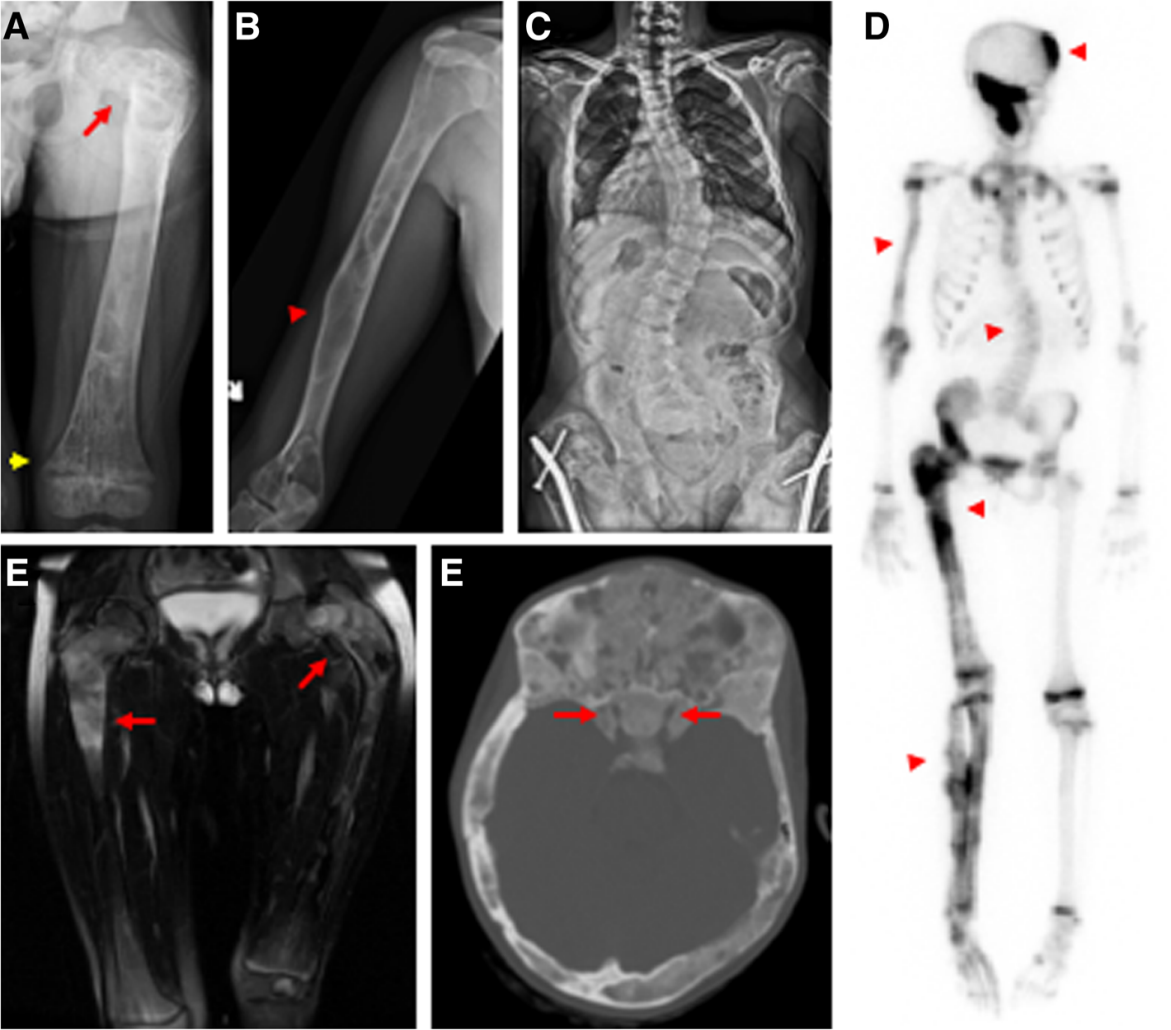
Representative radiographic features of fibrous dysplasia. a Femoral X-ray demonstrating diffuse involvement with fibrous dysplasia and a coxa vara (“shepherd’s crook”) deformity (red arrow). Note the irregular appearance of the distal femoral metaphyses (yellow arrowhead) resulting from FGF-23-mediated rickets. b Humeral X-ray demonstrating characteristic features of fibrous dysplasia, including homogenous “ground glass” appearance and cortical thinning. Bowing has occurred at a previously fractured site in the midshaft (red arrowhead). c X-ray from a patient with diffuse spinal FD and resulting thoraco-lumbar scoliosis. Note the presence of bilateral intramedullary femoral rods. d Technetium-99 scintigraphy scan showing increased tracer uptake in areas of fibrous dysplasia, including the skull, spine, right humerus, and right lower extremity (red arrowheads). Diffuse bilateral tracer uptake is also observed in the epiphyses of this growing adolescent. e T2-weight magnetic resonance imaging of the lower extremities showing well-demarcated lesions of intermediate to high signal intensity in the bilateral femurs (red arrows), corresponding to fibrous dysplasia lesions. f Computed tomography of the skull showing diffuse homogenous, “ground glass” involvement characteristic of craniofacial fibrous dysplasia. The bilateral optic canals are involved with fibrous dysplasia and widely patent (red arrows)
